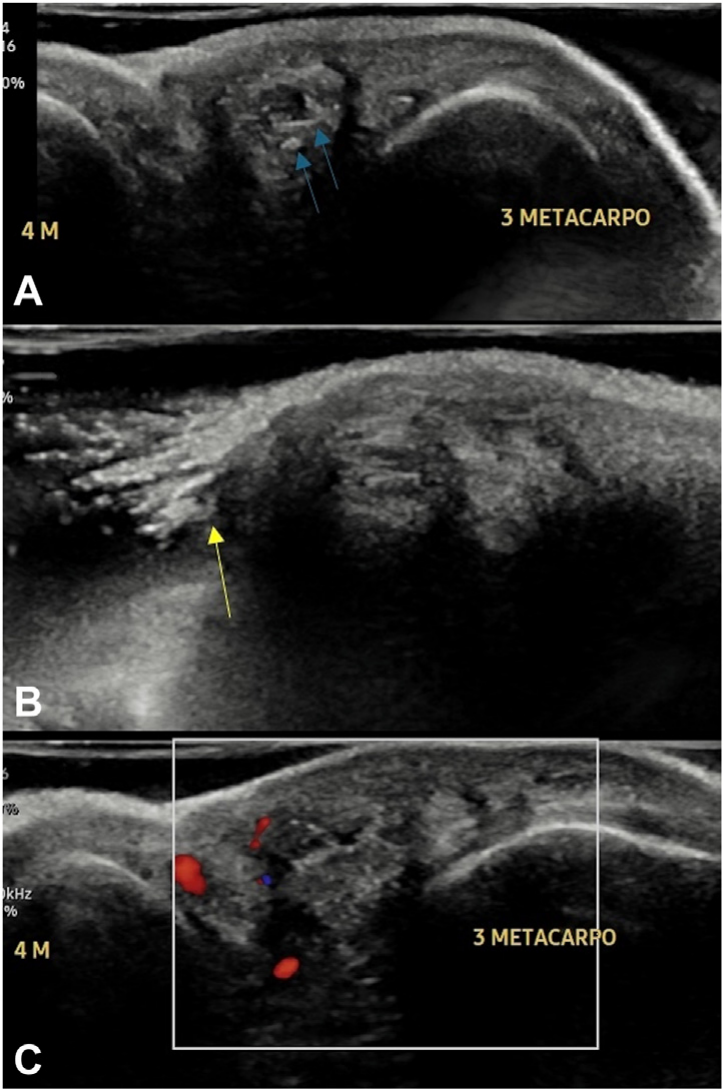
A, Ultrasound image showing a cross-section between the third and fourth left metacarpals, demonstrating a heterogeneous nodular formation with interspersed hyperechoic linear images (*blue arrows*) in the dermis and subcutaneous tissue; B, Longitudinal section showing the continuity of hyperechoic images through an area of discontinuity in the interdigital epidermis (corresponding to hair follicles), indicated by the *yellow arrow*. C, Doppler study of the lesion reveals predominantly peripheral vascularization.
